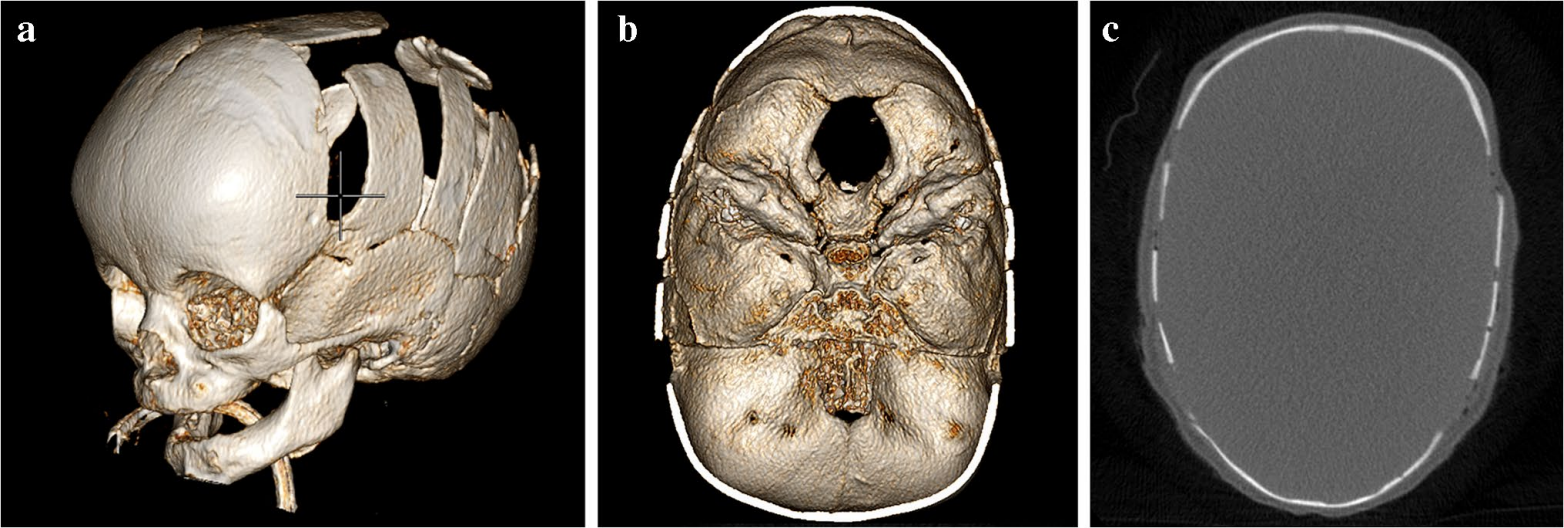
Postoperative non-contrast-enhanced cone-beam computed tomography (CBCT) images of a 5-month-old boy with sagittal craniosynostosis. Three-dimensional surface-rendered (a) intracranial surface-rendered (b) and axial (c) images clearly show the cranial sutures. However, the low-dose CBCT images did not have sufficient soft tissue contrast to show the ventricles
